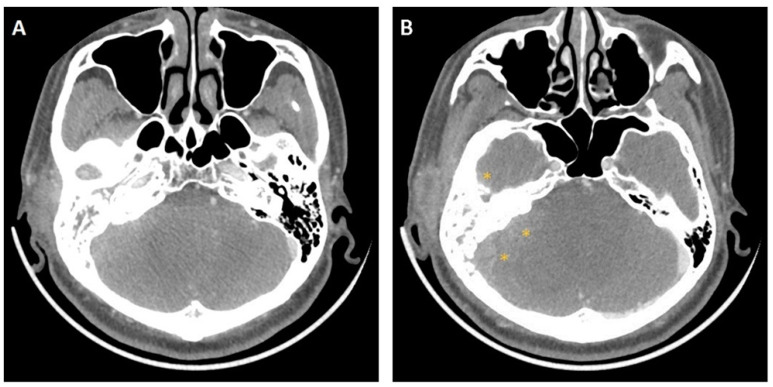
Case 4: High-resolution computed tomography scans show (A) infiltration of the soft tissue, with increased enhancement in the right mastoid and middle ear, and (B) intracranial extension to the right lateral areas of the posterior and middle cranial fossae (*).
